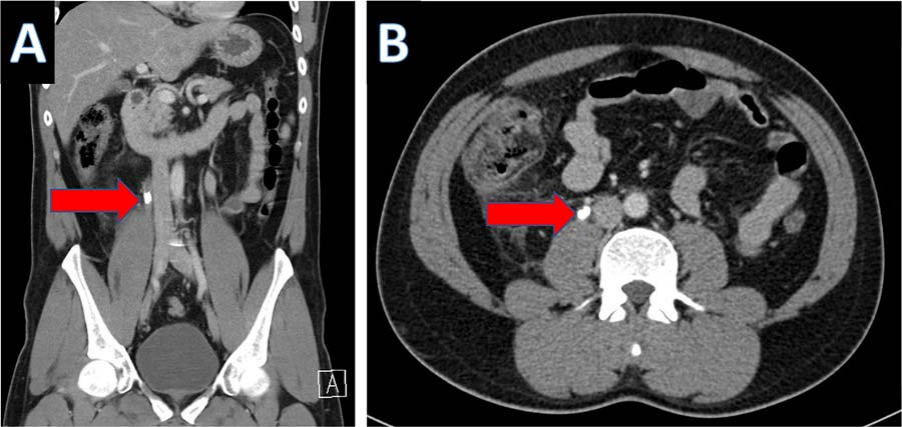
CT scan showing right ureteral calculus (A: coronal; B: axial).
